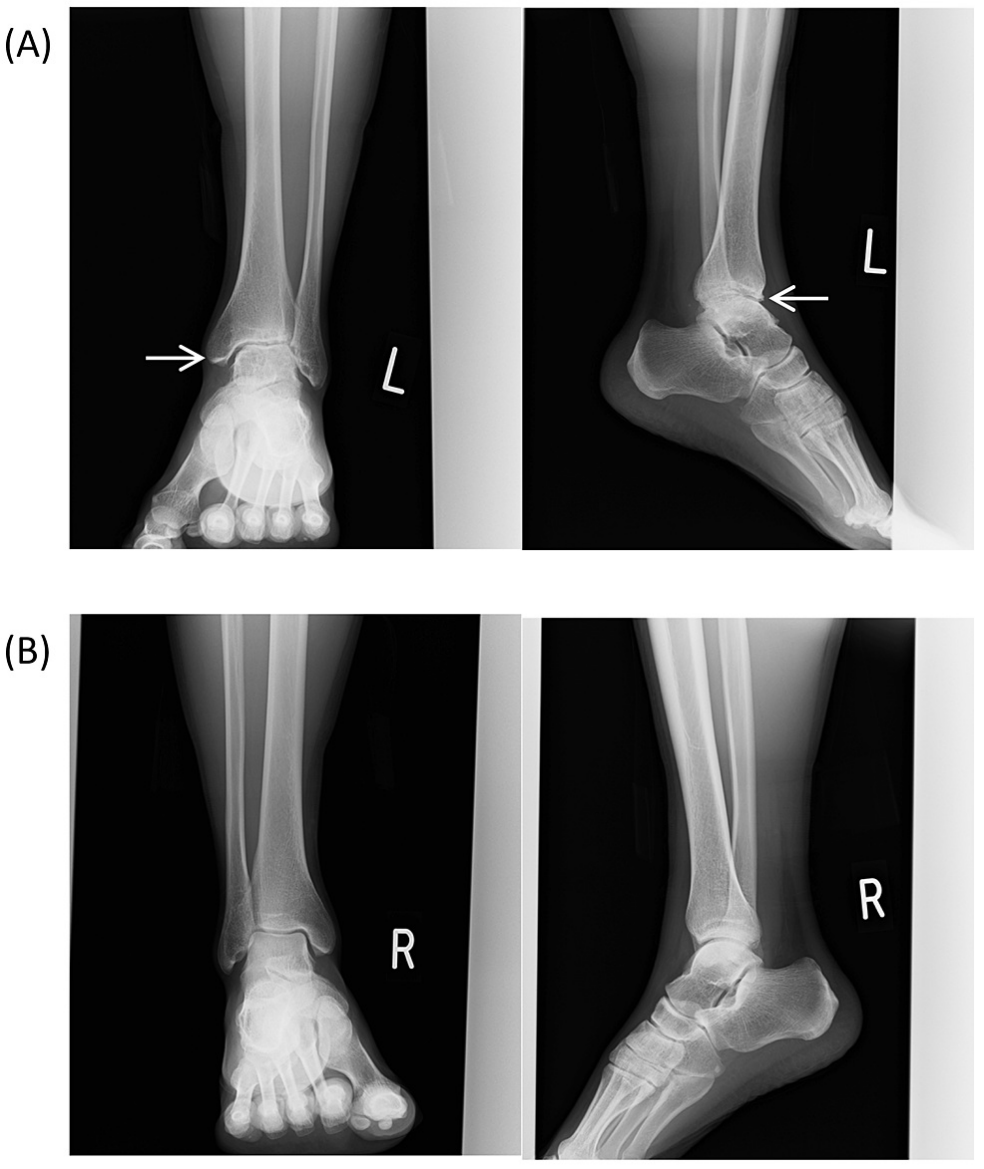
Joint X-ray images of Case 1 (A) left ankle joint showing advanced stage arthropathy (arrow) and (B) right ankle joint in September 2019 before albutrepenonacog alfa (rFIX-FP) treatment.
